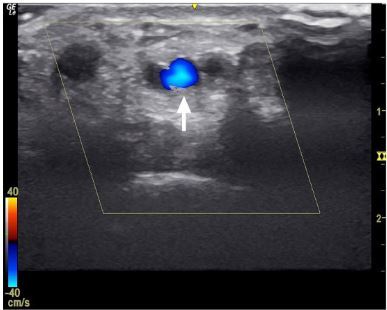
The image exhibits the ultrasound of the radial artery distal to the injury in transverse view. The white arrow demonstrates the radial artery, with color Doppler over the vessel. The blue color within the radial artery signifies retrograde flow, with blood flow in the direction away from the ultrasound probe.
